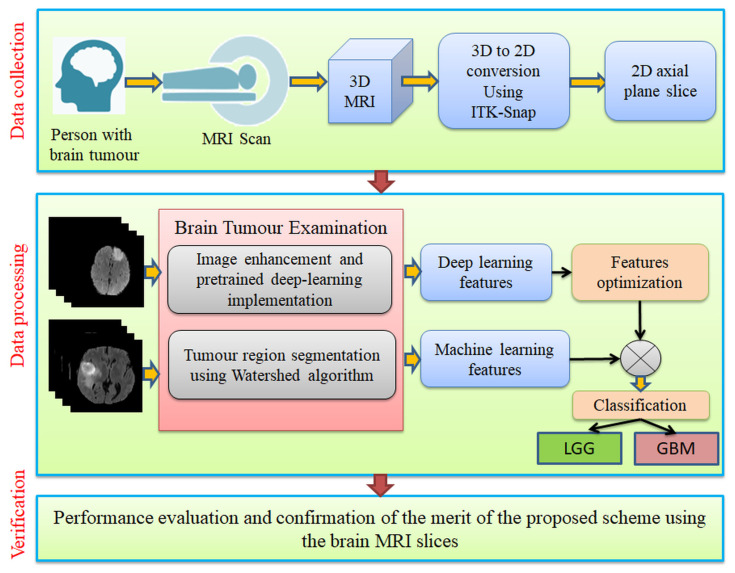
Structure of the proposed scheme to examine BT in MRI slices of FLAIR/T2 modalities with and without the skull.
